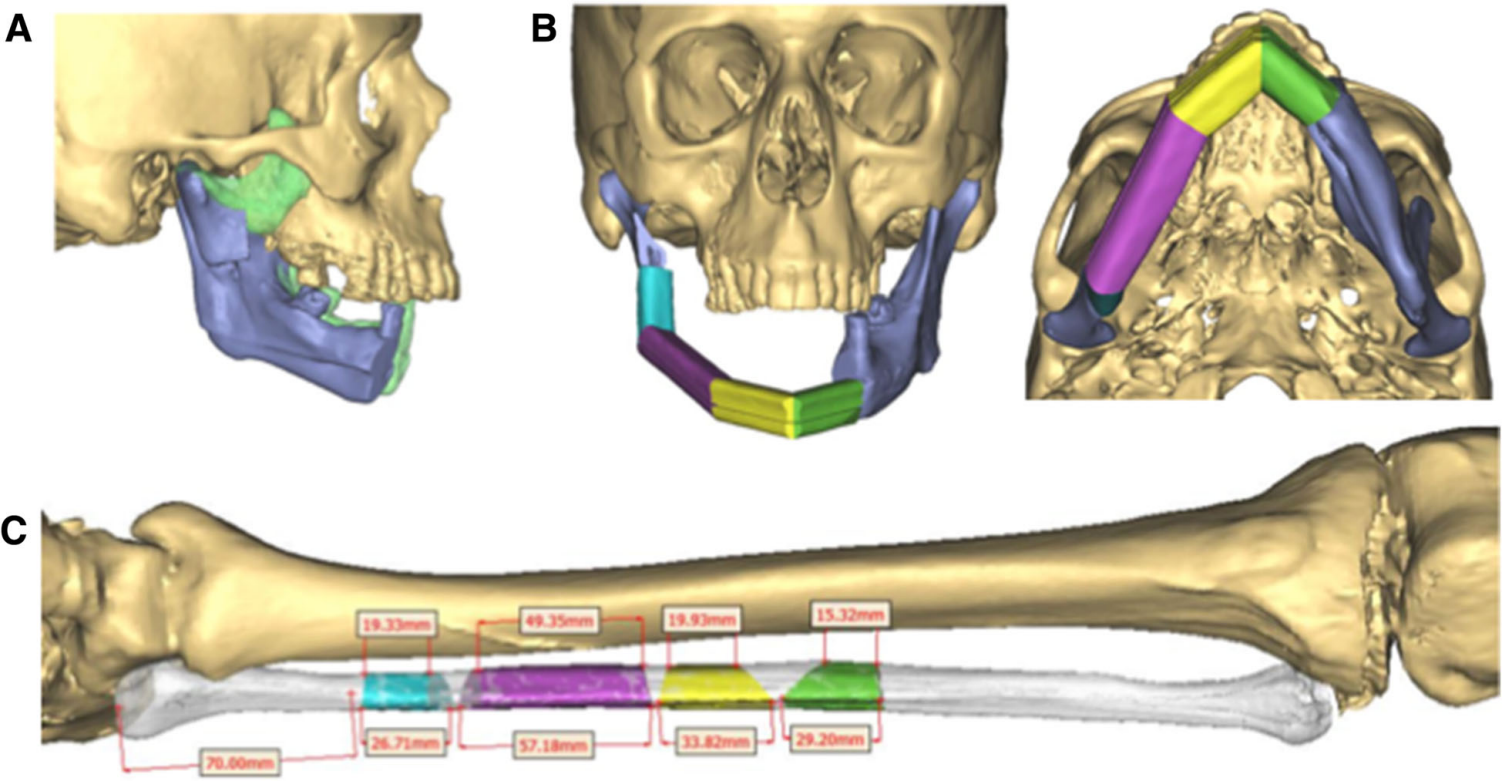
Planned reconstruction with 4 fibular segments. a 3-D CT reconstruction demonstrating resection of the remnant coronoid and repositioning of the right condyle. Original position shown in *green* with the planned reposition in *blue*
